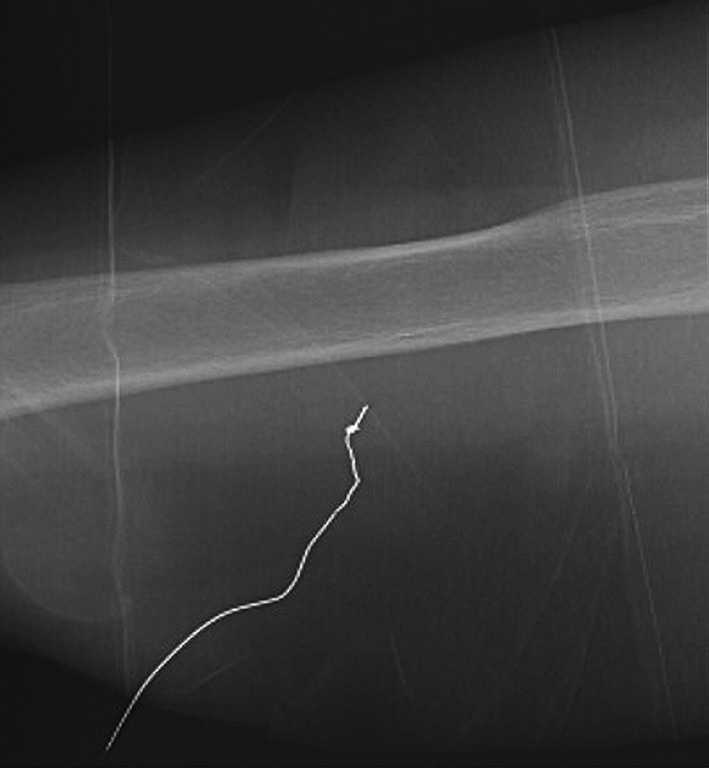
Radiograph indicating a knot‐like appearance at the tip of the guidewire
